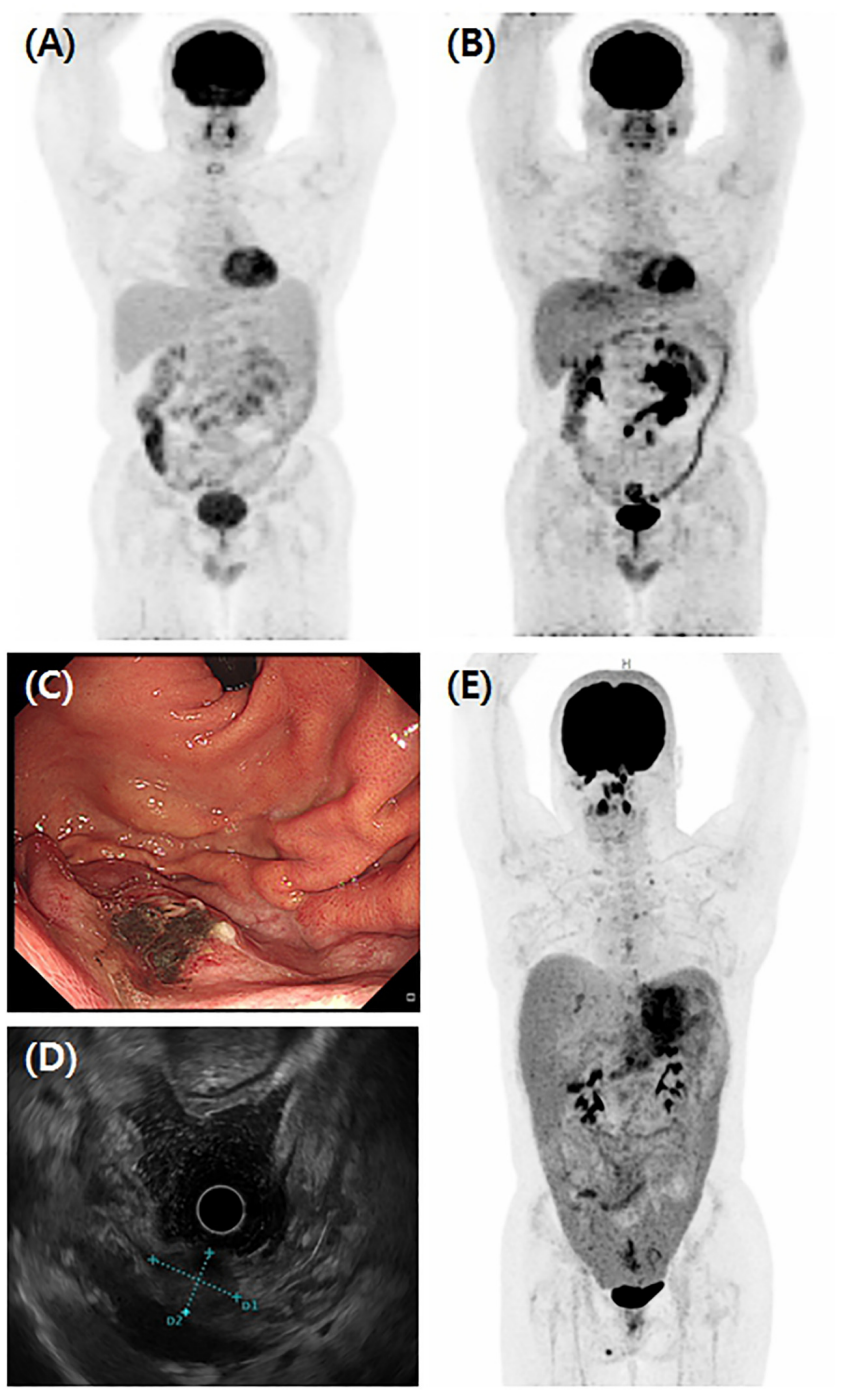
Various clinical manifestations of HS. (A) Initial findings of Case 1 FDG-PET/CT showed lymphoproliferative disease involving terminal ileum, ascending colon, and extensively throughout small bowel mesentery concurrent with lymph nodes in right retrocrural, aortocaval, retrocaval, and left paraaortic areas. This patient was diagnosed with follicular lymphoma and received six cycles of BR regimen. However, (B) follow-up FDG-PET/CT showed further increased activity of mass in mesentery and excision biopsy of mass diagnosed as HS. In Case 9, (C) the gastroscopic finding showed a flat elevated mass located on the high body of greater curvature, measuring about 2 cm in size. (D) Endoscopic ultrasound showed a 21 x 15 mm sized ovoid shape heterogeneous, hypoechoic mass with an ill-defined smooth border. (E) FDG-PET/CT of Case 9 showed gastric subepithelial tumor infiltrating the perigastric area and strong FDG uptakes in the peritoneum, omentum, and mesentery combined with upper abdominal, paraaortic, both iliac, and both cervical lymph nodes enlargements.
